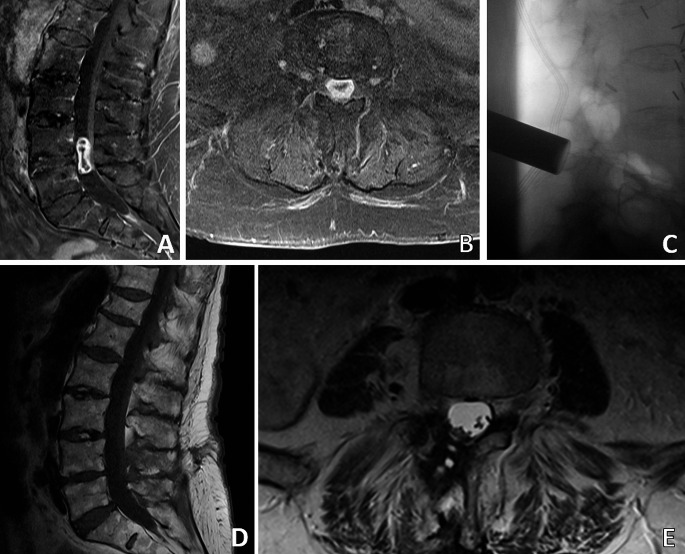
Exemplary case of an Ia classified intraspinal, intradural schwannoma (case 46). Sagittal (A) and axial (B) preoperative MRI scans show the lesion. The intraoperative X-ray shows the placement of the tubular retractor (C). Postoperative findings of case 46: Sagittal (Dt) and axial (Et) MRI scans show GTR
